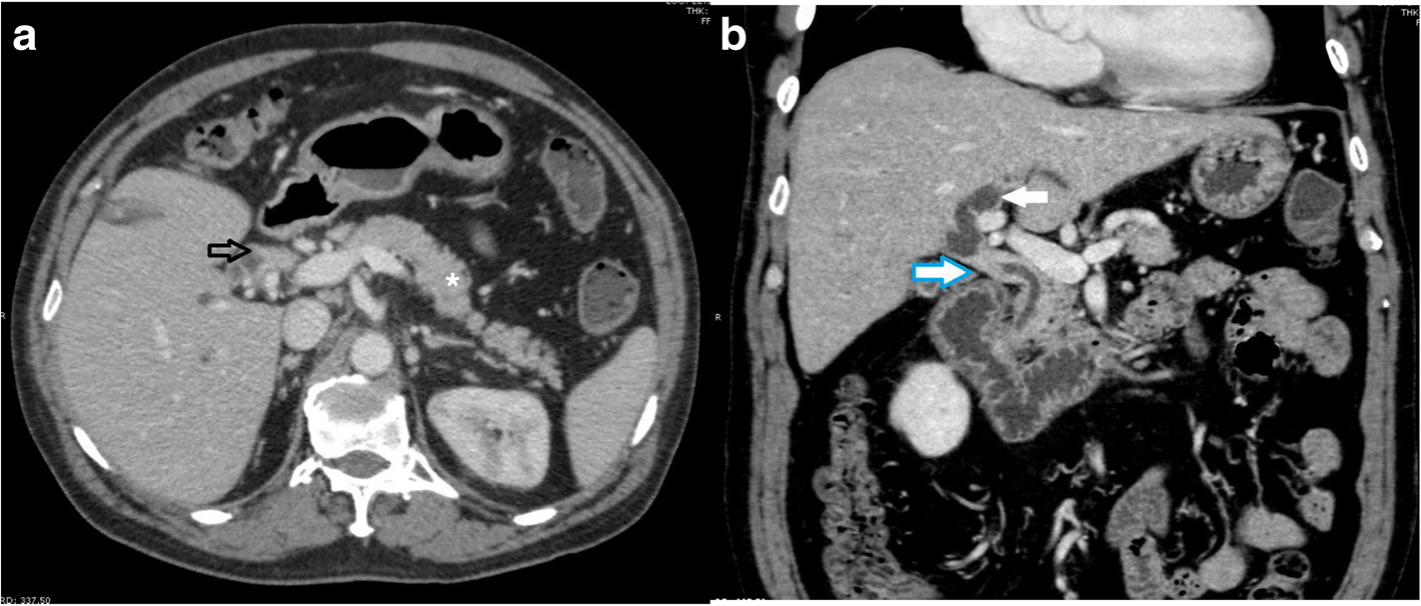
Abdominal CT showing focal short segment sheet-like thickening of proximal common bile duct with enhancement. a: axial view showing sheet-like thickening of common hepatic duct wall (*arrow*); note the normal pancreas (*star*). b: coronal view showing focal short segment, sheet-like, symmetric thickening of common hepatic duct causing nearly complete obstruction of common hepatic duct just below bifurcation (*white arrow with blue edge*) of right and left intrahepatic bile ducts and dilatation of left intrahepatic duct (*white arrow*)
